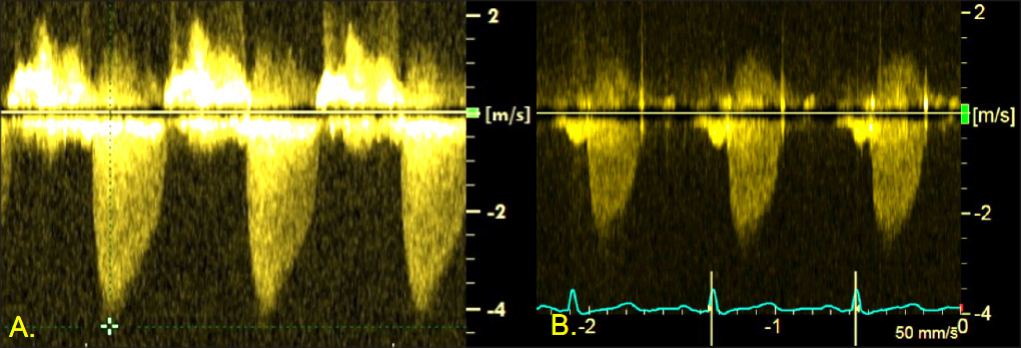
Doppler echocardiography of stenotic aortic valve. (A) Baseline severe aortic stenosis. (B) Follow-up post-procedure echocardiography.
